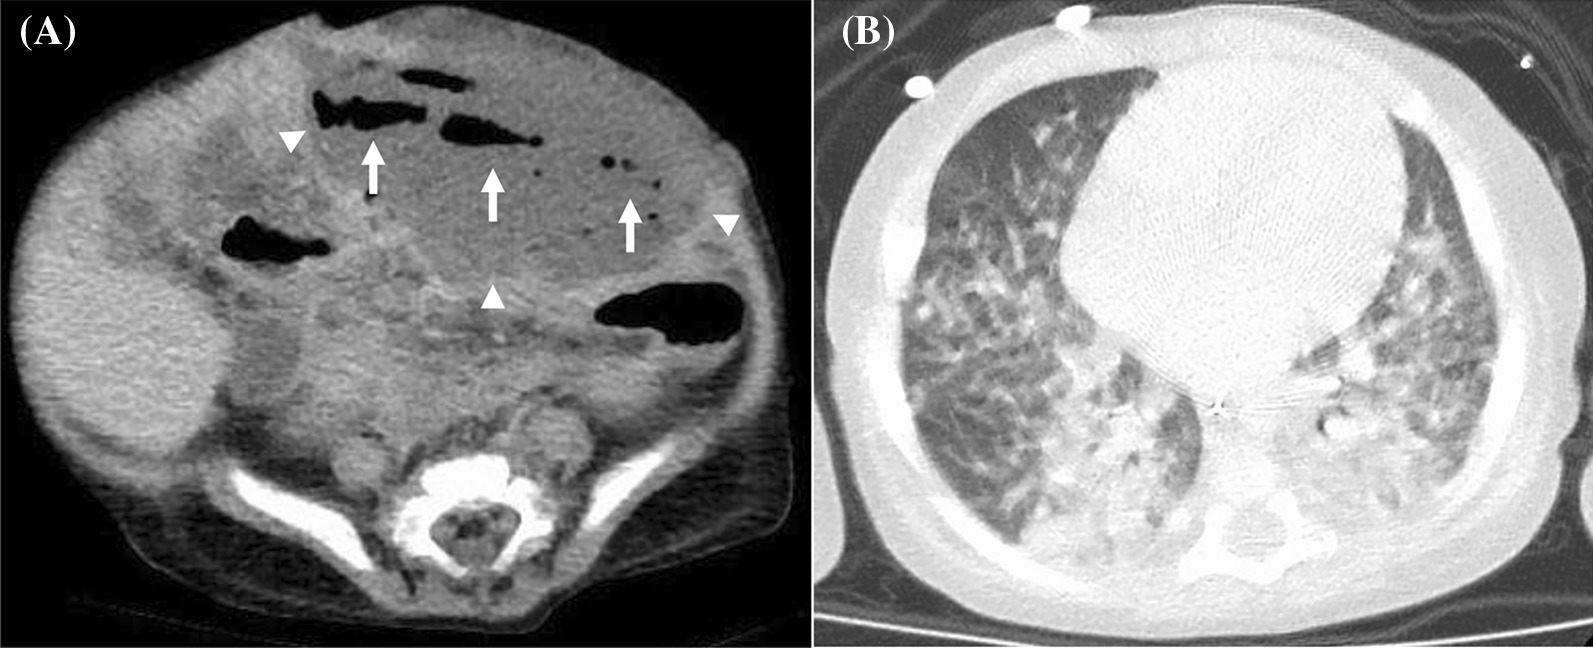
A Contrast-enhanced abdominal computed tomography showing extensive irregular necrotic mass (arrowheads) with gas (arrows), connecting with the small intestine and subcutaneous tissue. B Chest CT showing consolidations and multiple nodules in both lungs
